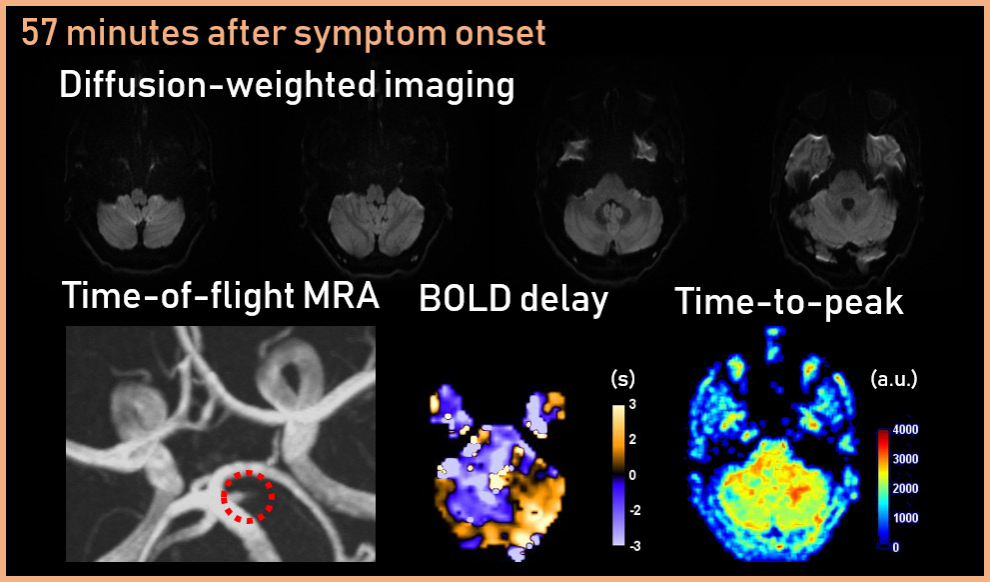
Baseline MRI showed no signs of intracranial haemorrhage or intracerebral restricted diffusion. Time-of-flight magnetic resonance angiography (TOF-MRA) showed left superior cerebellar artery (SCA) occlusion. The BOLD delay and time-to-peak maps showed an area of delayed blood flow in the portion of the left cerebellar hemisphere supplied by the SCA.
